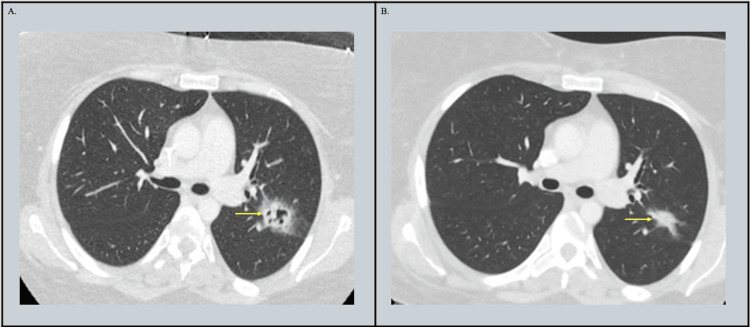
(A) Initial chest CT at admission showing cavitary lesion in the left upper lobe. (B) CT after 27 days of levofloxacin showing resolution of cavitation.
